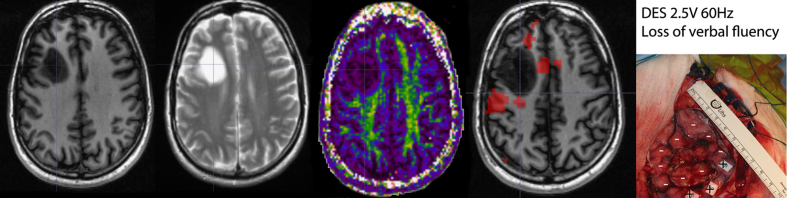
From left to right, the first 3 images show structural data: T1-weighted, T2-weighted, Diffusion weighted (Fractional Anisotropy map here) images. These data are complemented by functional BOLD imaging and Direct Electrical Stimulation.
